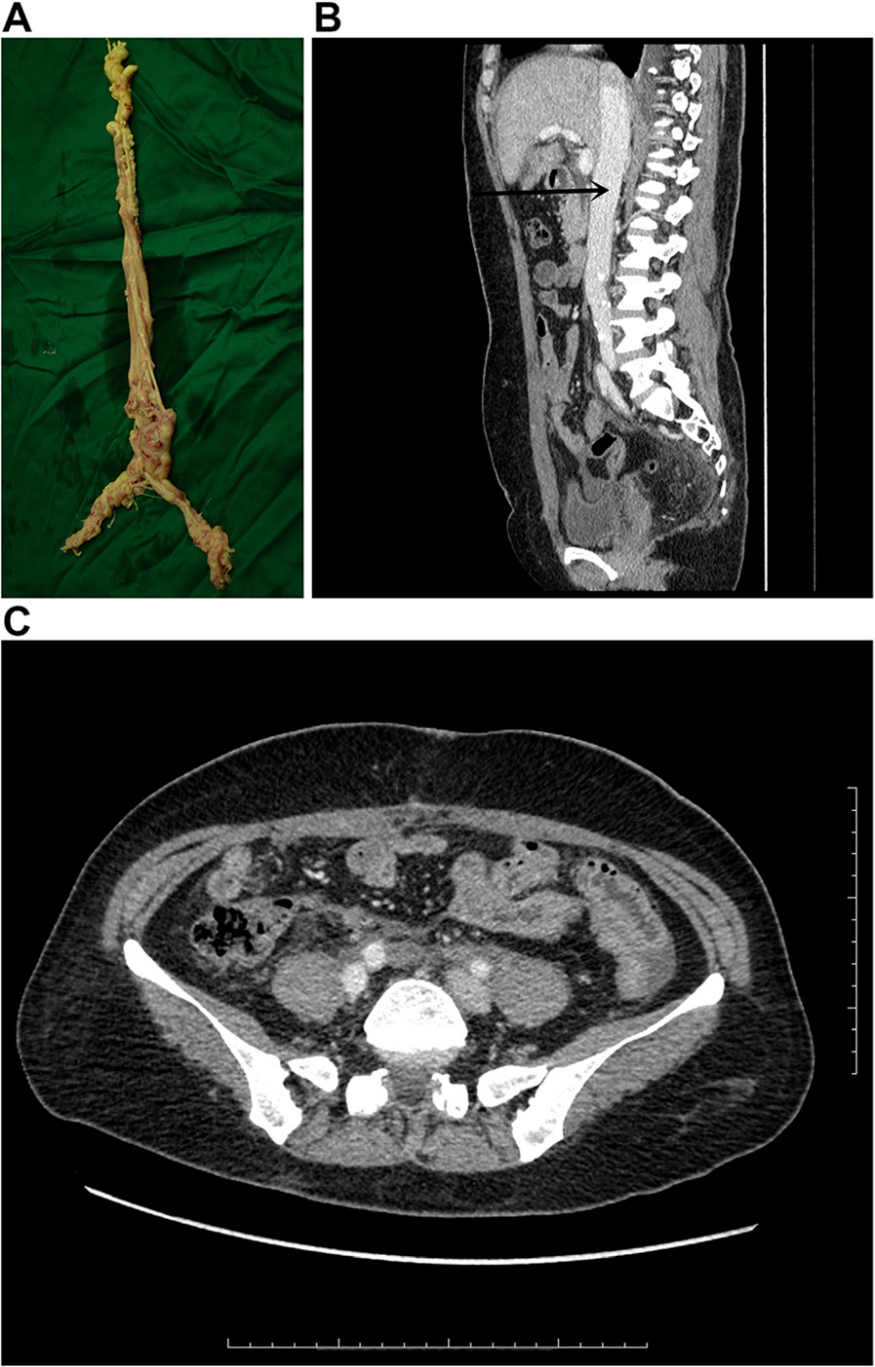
The tumor in the double common iliac veins, IVC, and heart was resected (a). The CT after operation showed complete resection of the tumor (b, c)
